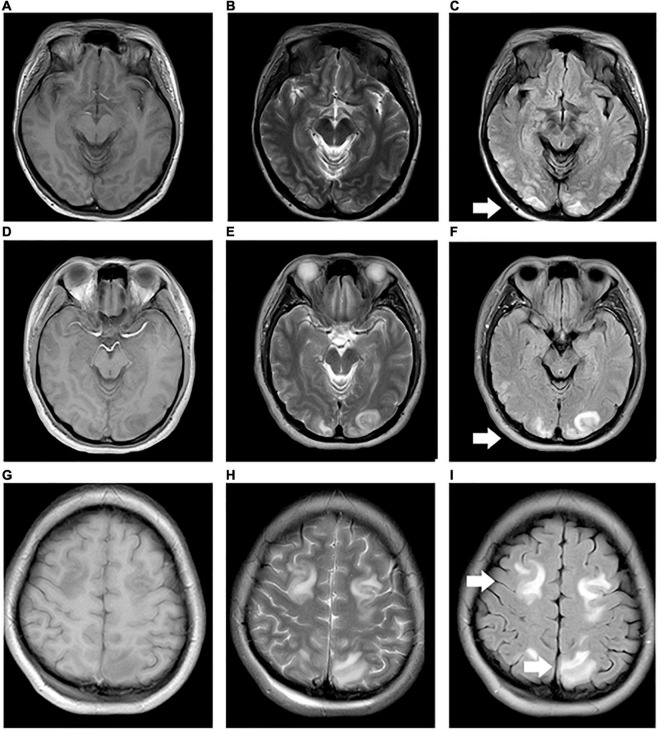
Maternal preversible encephalopathy syndrome. (A–I) Maps showing 33, 28, and 24 year-old pregnant women (BH: 200/130, 160/110, and 180/110 mmHg) with decreased signal intensity on T_1_WI, and increased signal intensity on T_2_WI and FLAIR in bilateral occipital regions, bilateral occipital and right temporal regions, and bilateral parietal and frontal regions, respectively.
